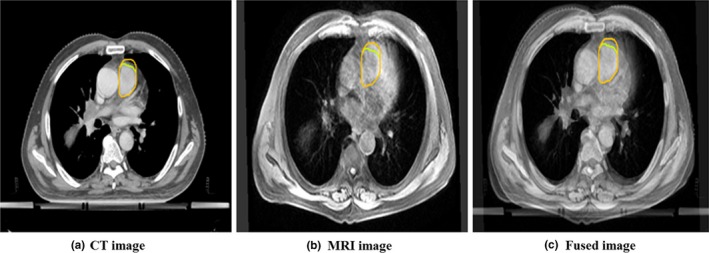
Image registration based on the pulmonary artery. (a) CT image, (b) MRI image, and (c) fused image.
